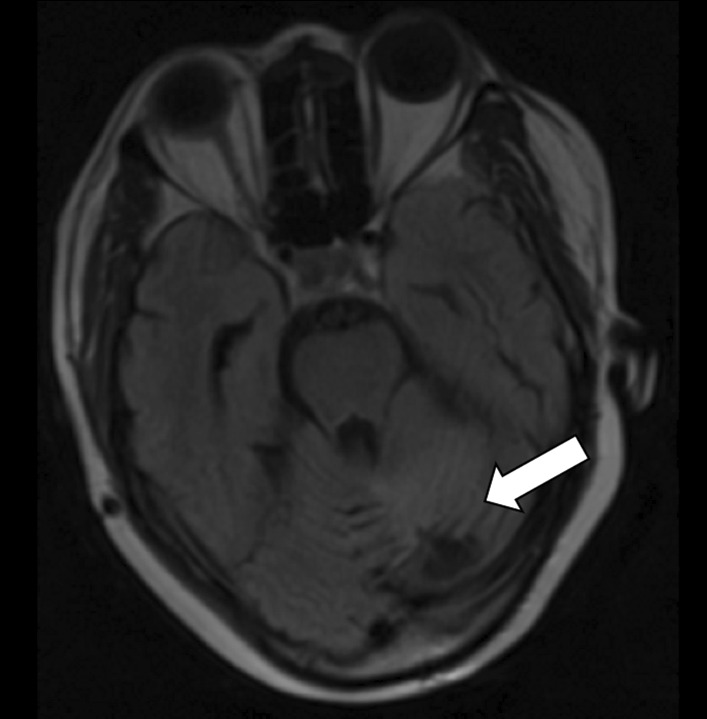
T1W brain MRI 9 months after finishing treatment showing a significant reduction in the posterior cranial fossa lesion as indicated by the arrow
